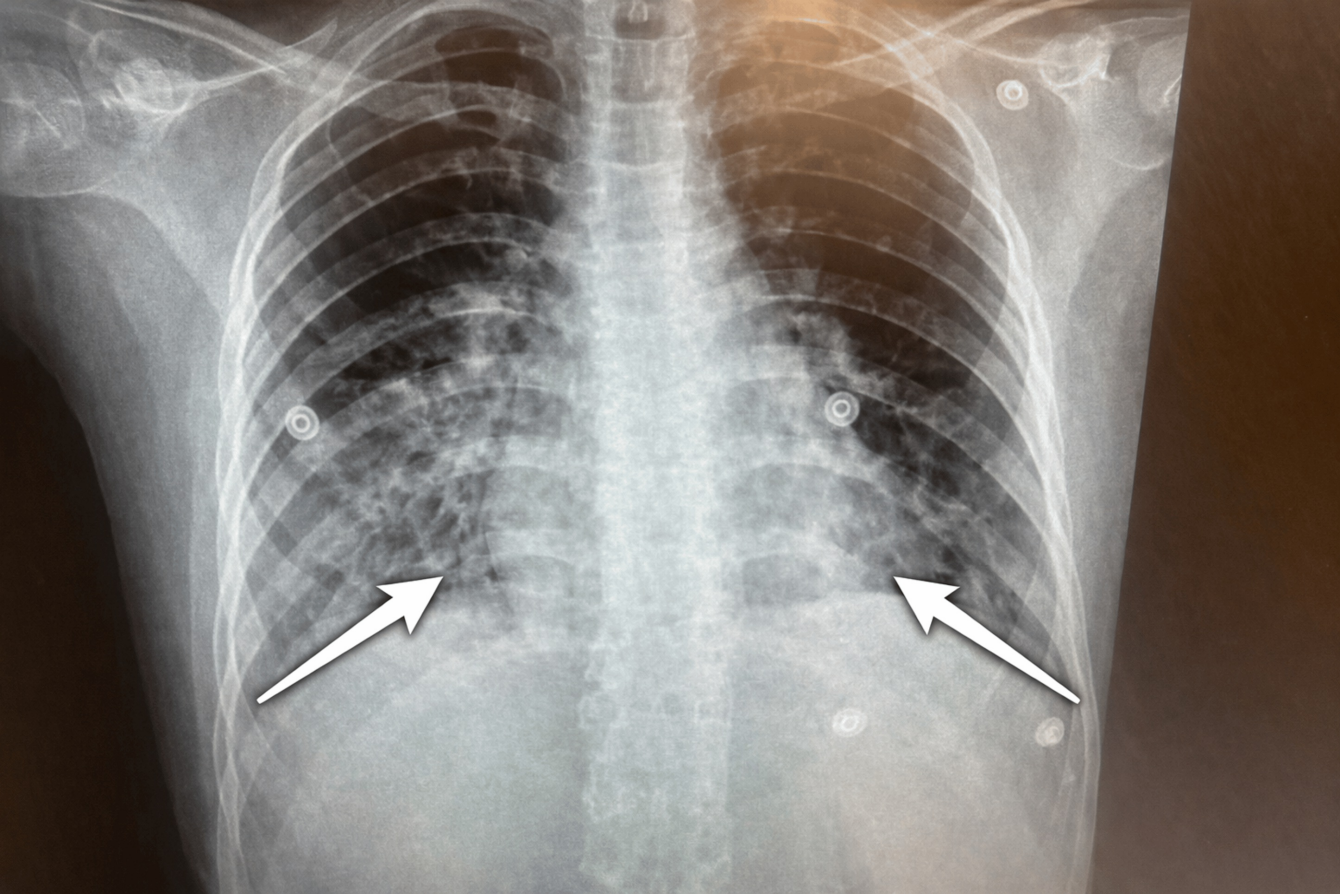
Chest radiograph demonstrating bilateral basal infiltrates (white arrows)
